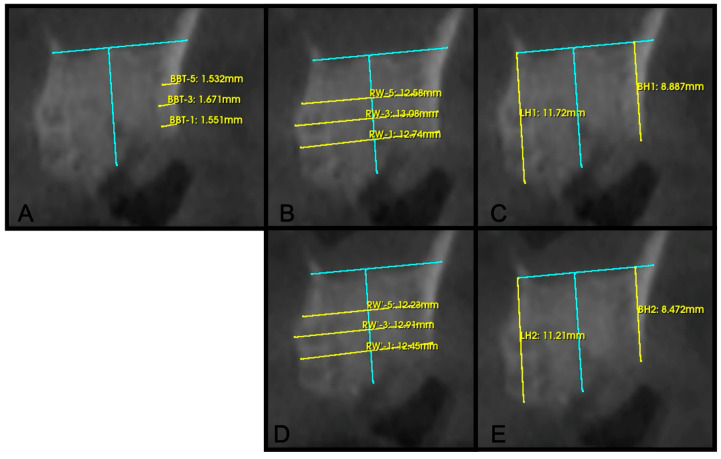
An example case of ridge preservation at site #15, demonstrating radiographic measurements. Immediate post-operative CBCT scan with: (A) Baseline buccal bone thickness at 1 mm (BBT-1), 3 mm (BBT-3), and 5 mm (BBT-5) apical to the crest; (B) Baseline measurements of ridge width at 1 mm (RW-1), 3 mm (RW-3), and 5 mm (RW-5) apical to the buccal crest; (C) Baseline measurements of Buccal height (BH) and Lingual Height (LH); (D) 3-month post-operative ridge width measurements at all levels; and (E) 3-month post-operative buccal and lingual height measurements. All measurements were taken with the same vertical and horizontal reference lines (in blue) at both time intervals.
